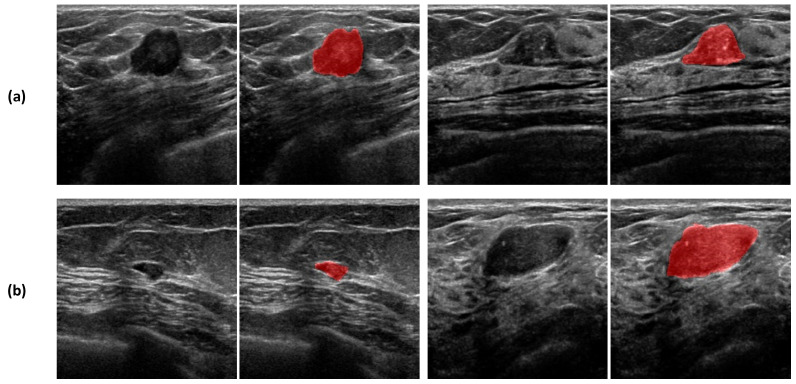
Samples of breast ultrasound images. (a) Malignant cases and (b) benign cases. Red colored-regions are breast lesions.
